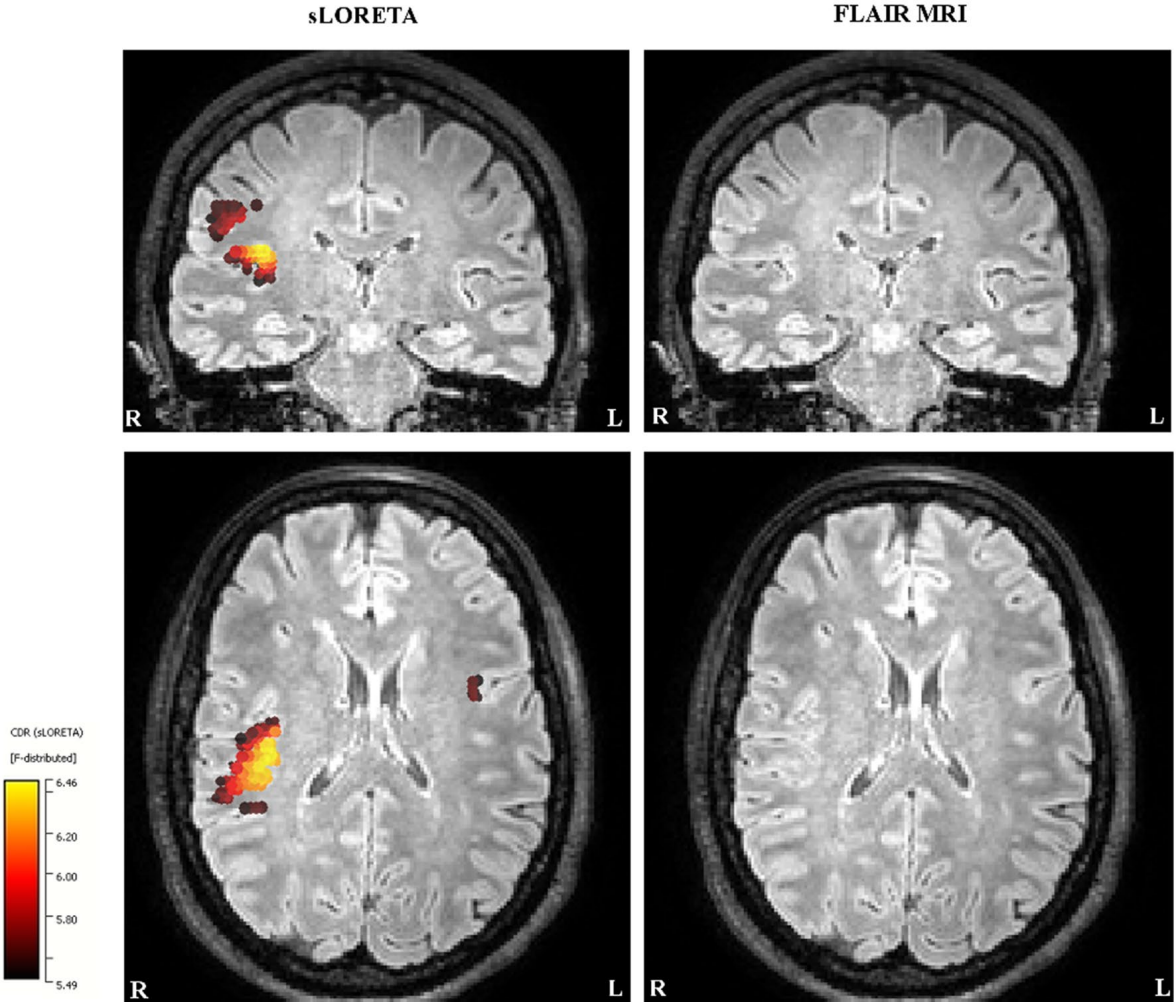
EMEG source localizations at −23 ms, slices selected according to the right hemispheric activity. The sLORETA results registered to the FLAIR MRI are shown in the left column (only results obtained with a threshold of 85% for the maximum F-value are shown). In the right column, the FLAIR image without the localizations is shown. The *white letters* on MRIs indicate the direction (*L* left, *R* right, *A* anterior, *P* posterior)
